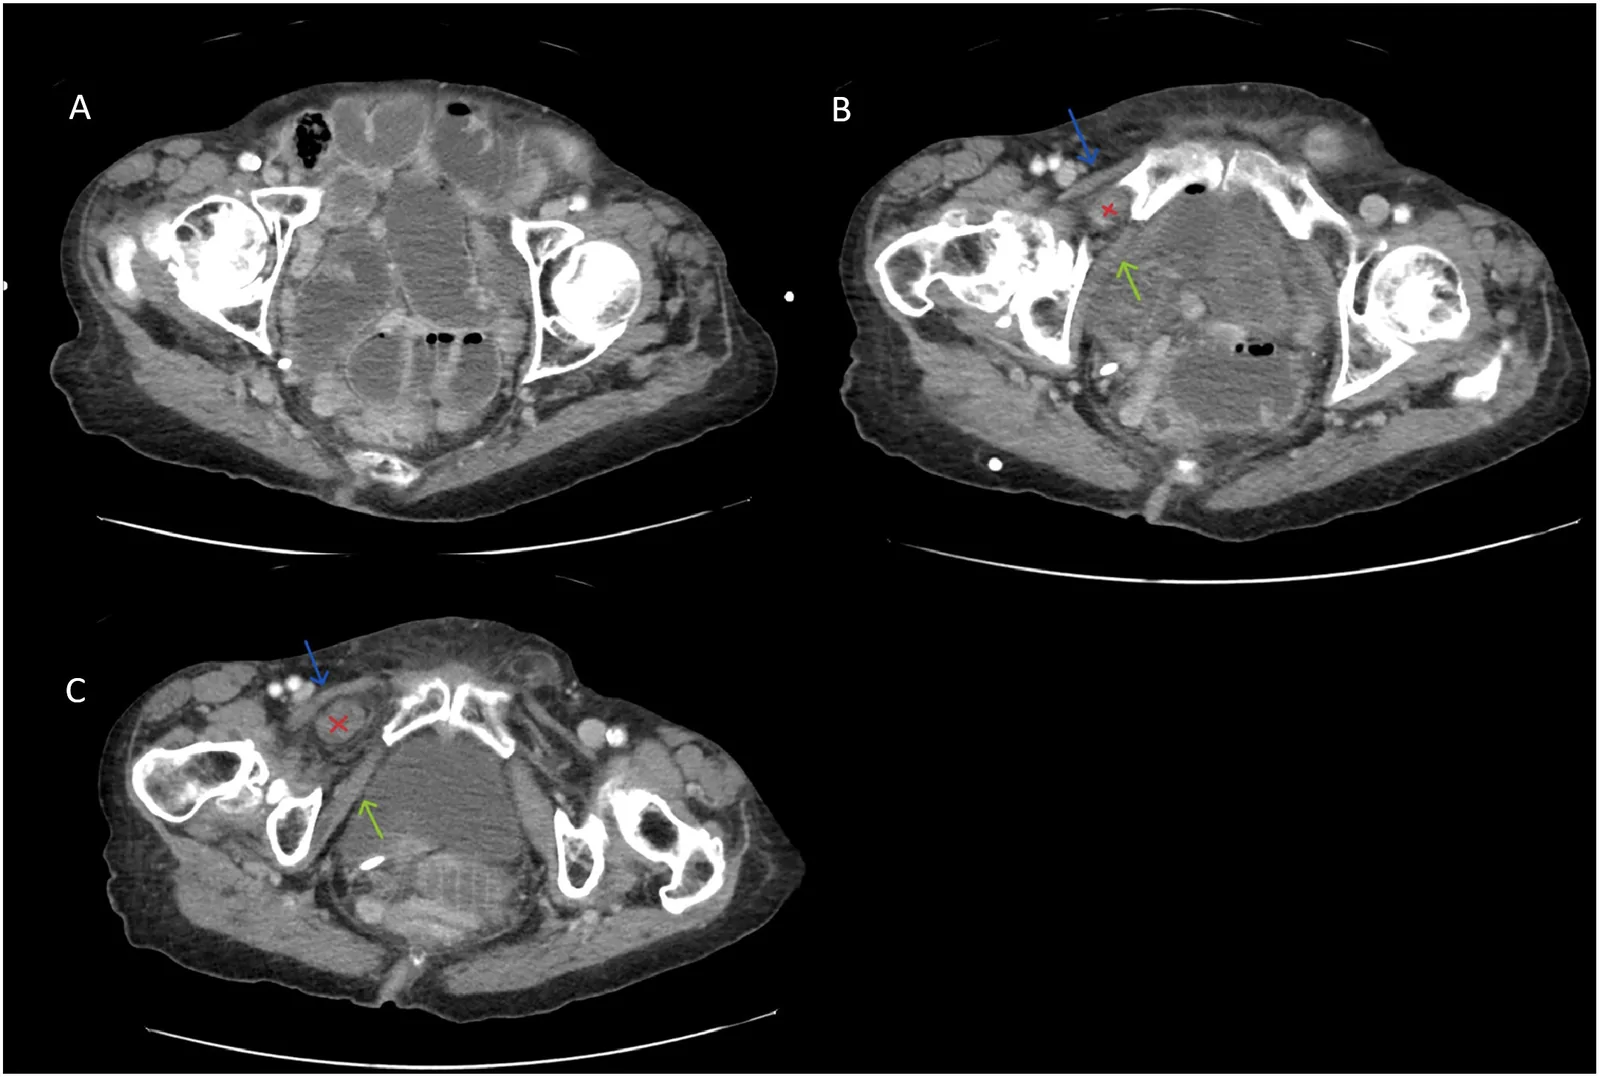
Sequential axial images from contrast-enhanced CT of the abdomen and pelvis demonstrate: (A) dilated loops of small bowel in the pelvis; (B) and (C) loop of small bowel (red X) interposed between the pectineus (blue arrow) and obturator musculature (green arrow). The obturator canal is widened and measures approximately 2.5 cm.
